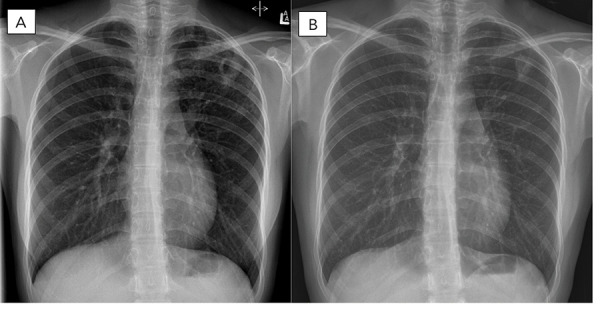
Chest X-ray (posterior-anterior film)^a^ ^a^ The left X-ray showing left upper lung thick walled cavitary lesion pretreatment with the right X-ray showing improvement on follow-up six-month post completion of treatment
